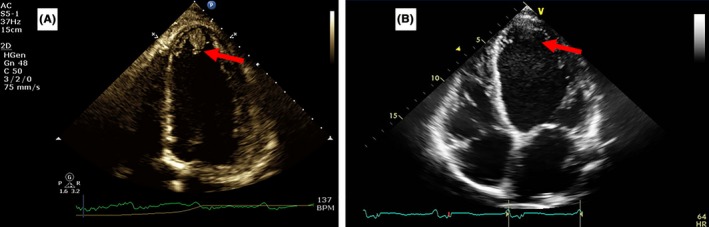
A, Apical four chamber view showing the LV thrombus of case five. B, Resolution of the thrombus after four months of anticoagulation with Apixaban
